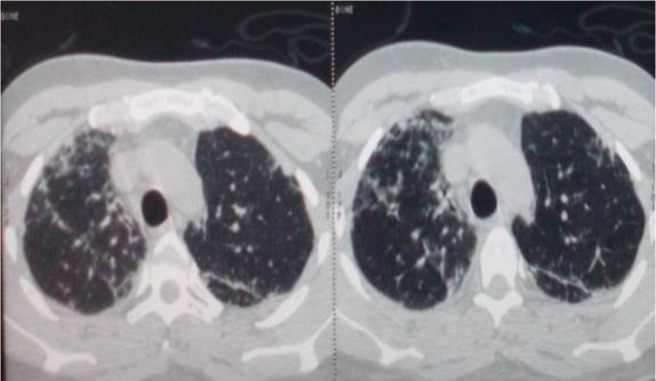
Chest High-resolution computed tomography showing bilateral micronodular opacities, traction bronchiectasis, thickening of septal lines, and a localized ground-glass opacities.
